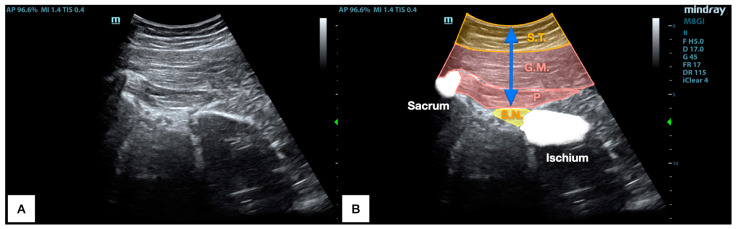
Raw ultrasound image acquired (A) and labeled image, highlighting the main structures (B). Blue arrow: distance between the skin and the sciatic nerve; G.M.: gluteus maximus; P: piriformis muscle; S.T.: subcutaneous tissue.
